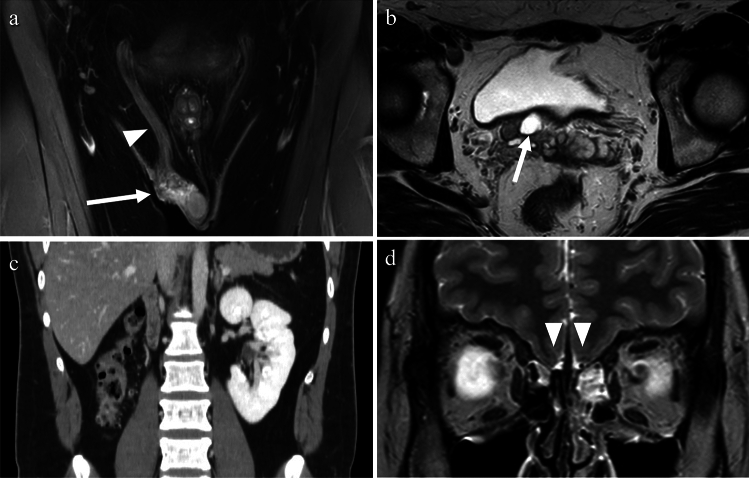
Man in his 20 s with Zinner and Kallmann syndrome presenting with recurrent right scrotal pain. a Fat-saturated contrast-enhanced T1-weighted image showing enlargement and enhancing effects in the right epididymis (arrow), with enhancement extending continuously along the spermatic cord (arrowhead), indicating epididymitis. b Cyst formation is also observed in the right seminal vesicle (arrow) on T2-weighted image. c Contrast-enhanced CT confirmed the right renal agenesis and a diagnosis of Zinner syndrome was made. d In addition, the absence of olfactory bulbs and olfactory grooves (arrowheads) on coronal T2-weighted image, combined with symptoms of hypogonadotropic hypogonadism and hyposmia, suggests Kallmann syndrome
